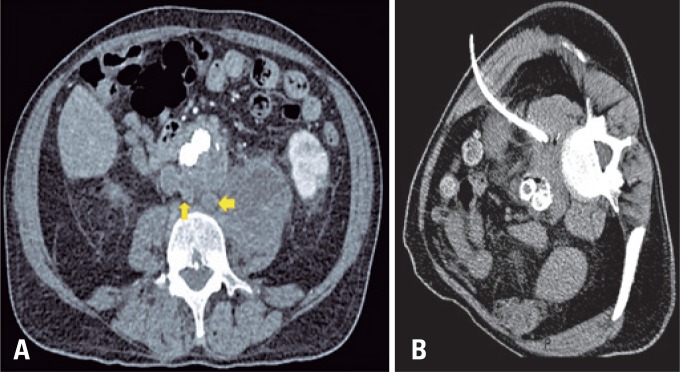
Percutaneous drainage of peri-prosthetic abscess. (A) Computed tomography angiography of the abdomen: aneurysm sac with heterogeneous fluid collection infiltrating the left psoas muscle, and reactive, enlarged retroperitoneal lymph nodes (arrows); (B) Computed tomography -guided drainage with placement of a 12F pigtail catheter
